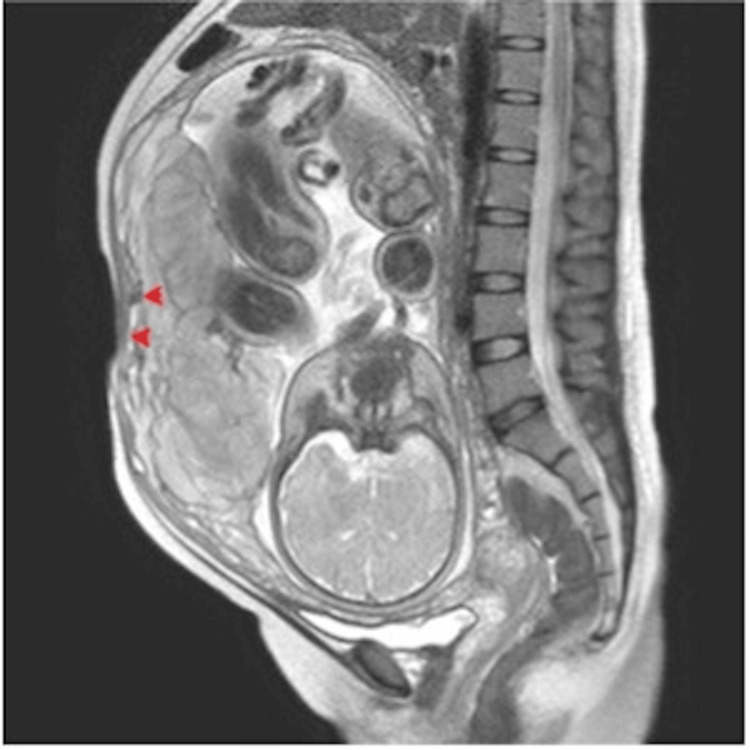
MRI showing placenta accreta (red arrowheads) with a fetus (Case 1).
